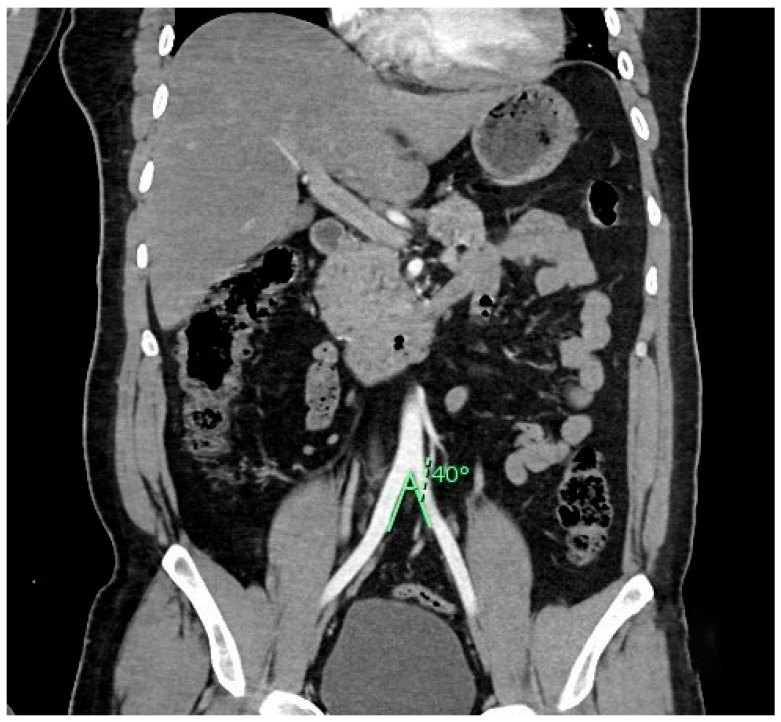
Coronal CT section showing the measurement of the AA bifurcation. AA: abdominal aorta.
